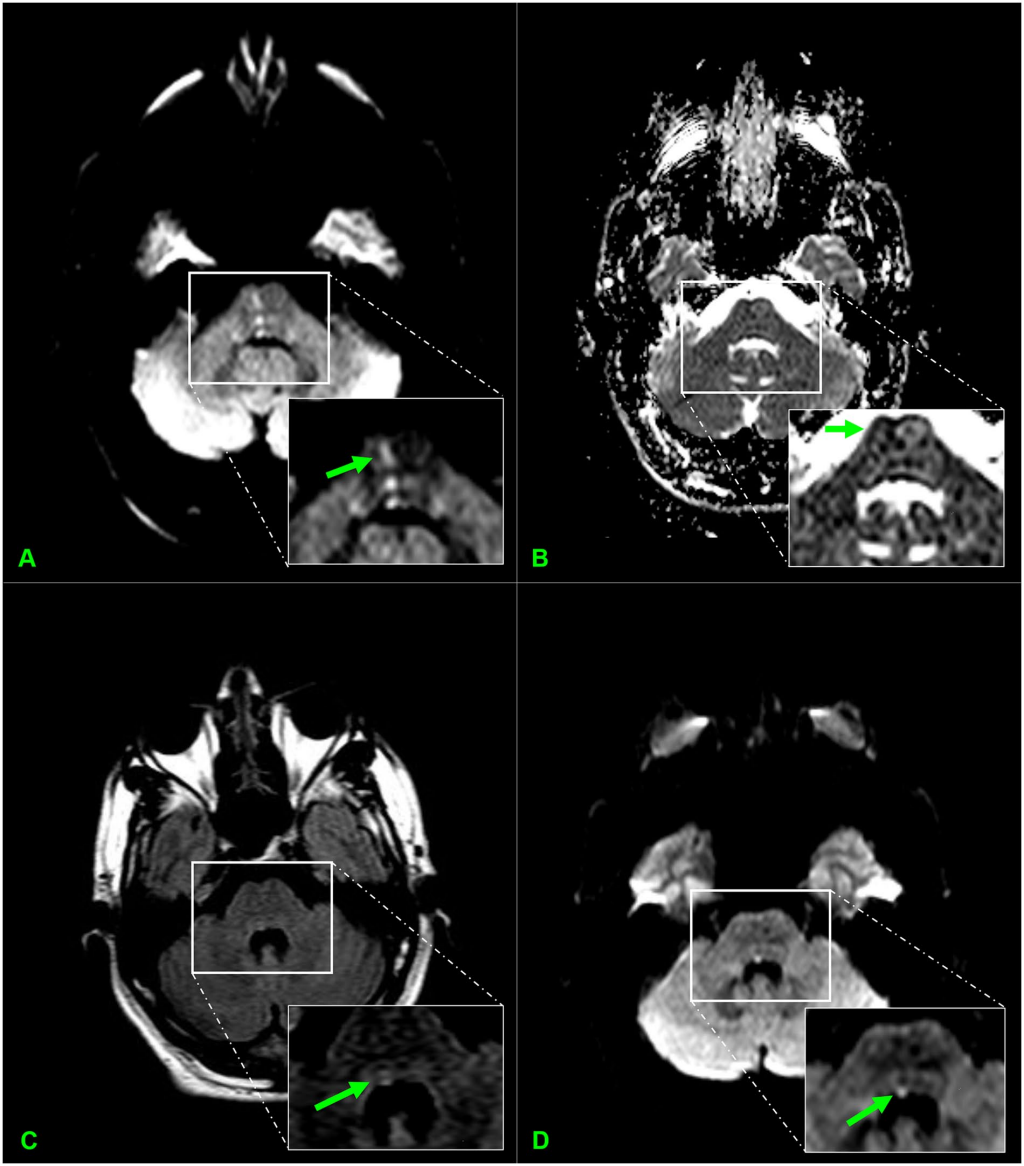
Different MRI sequences showing a subacute ischemic infarct (green arrows). (A) Diffusion-weighted imaging (DWI) showing hyperintensity in the right pons. (B) Apparent Diffusion Coefficient (ADC) showing hypointensity in the right pons. (C) T2 Fluid-Attenuated Inversion Recovery (FLAIR) showing hyperintensity in the right facial colliculus. (D) DWI showing hyperintensity in the right facial colliculus.
